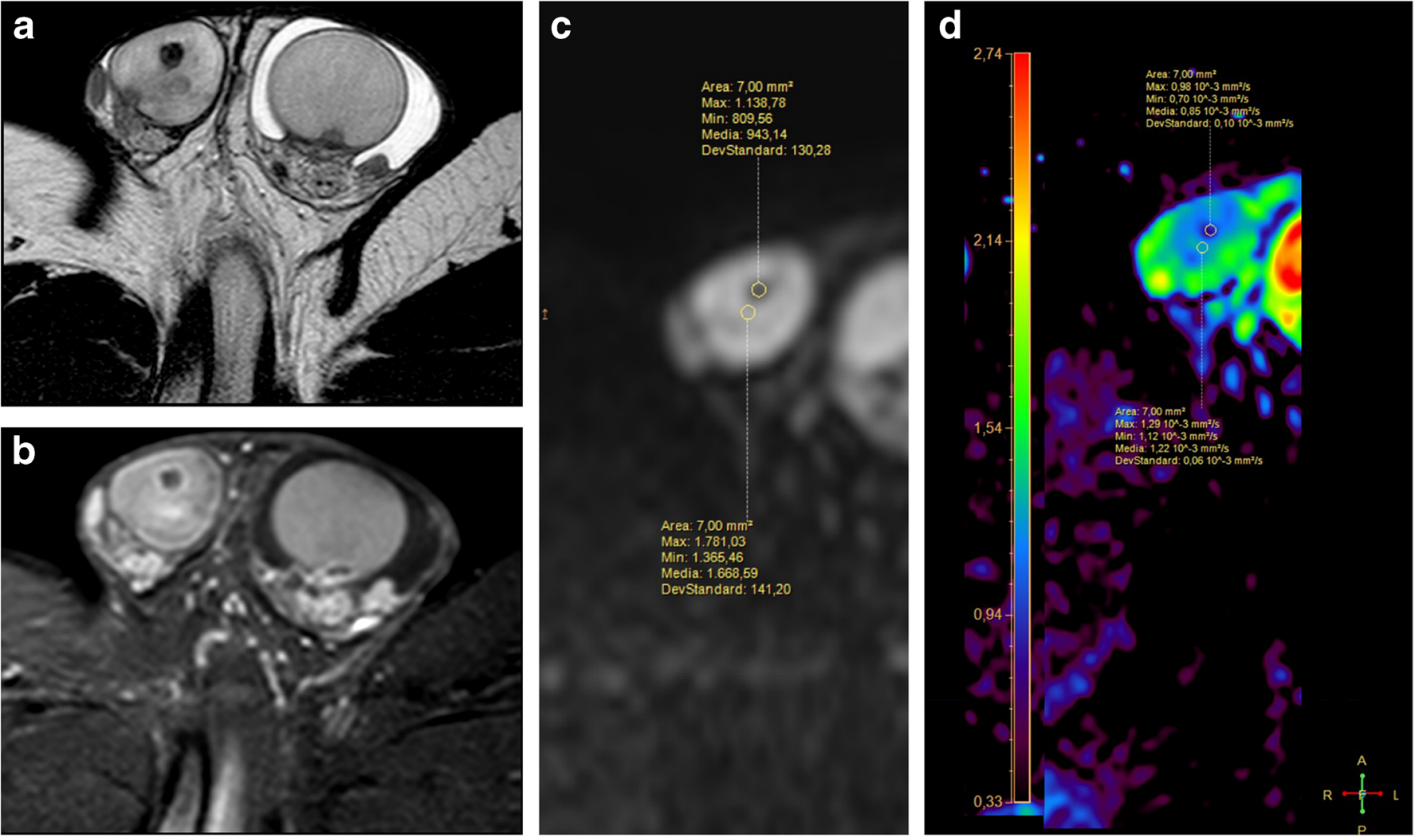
a-d MR study of a multifocal mixed embryonal carcinoma. a T2-weighted TSE sequence, axial view; b postcontrast T1-weighted SPIR TSE sequence.; c-d ADC
